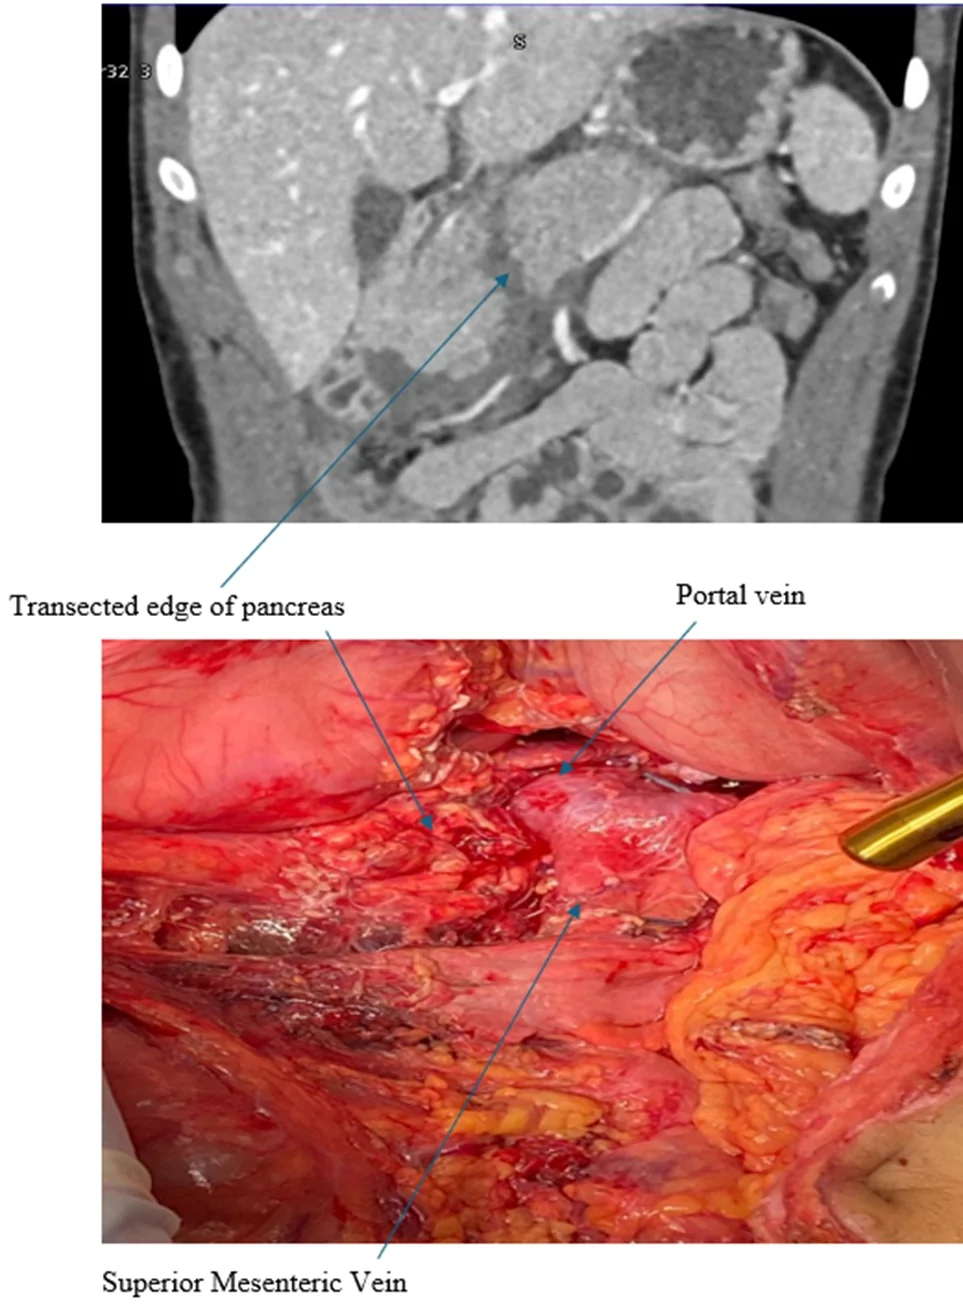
Grade III pancreatic injury (Upper: CT scan and Lower: intraoperative).
